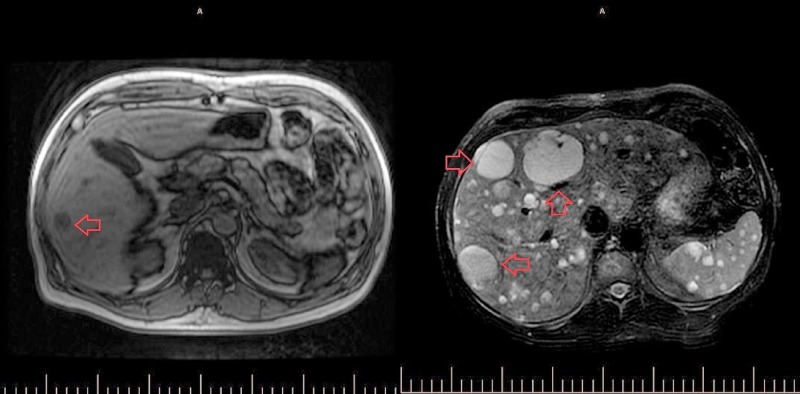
Side-by-side MRI abdomen and pelvis with and without contrast The study on the left was conducted at initial presentation and shows multiple liver lesions (small, dark gray lesions in the liver, the largest of which is highlighted with a red arrow). The follow-up MRI two months later revealed the progression of metastatic disease within the liver, spleen, and spine (light gray; the largest three lesions highlighted by the red arrows). MRI: magnetic resonance imaging; CT: computed tomography
